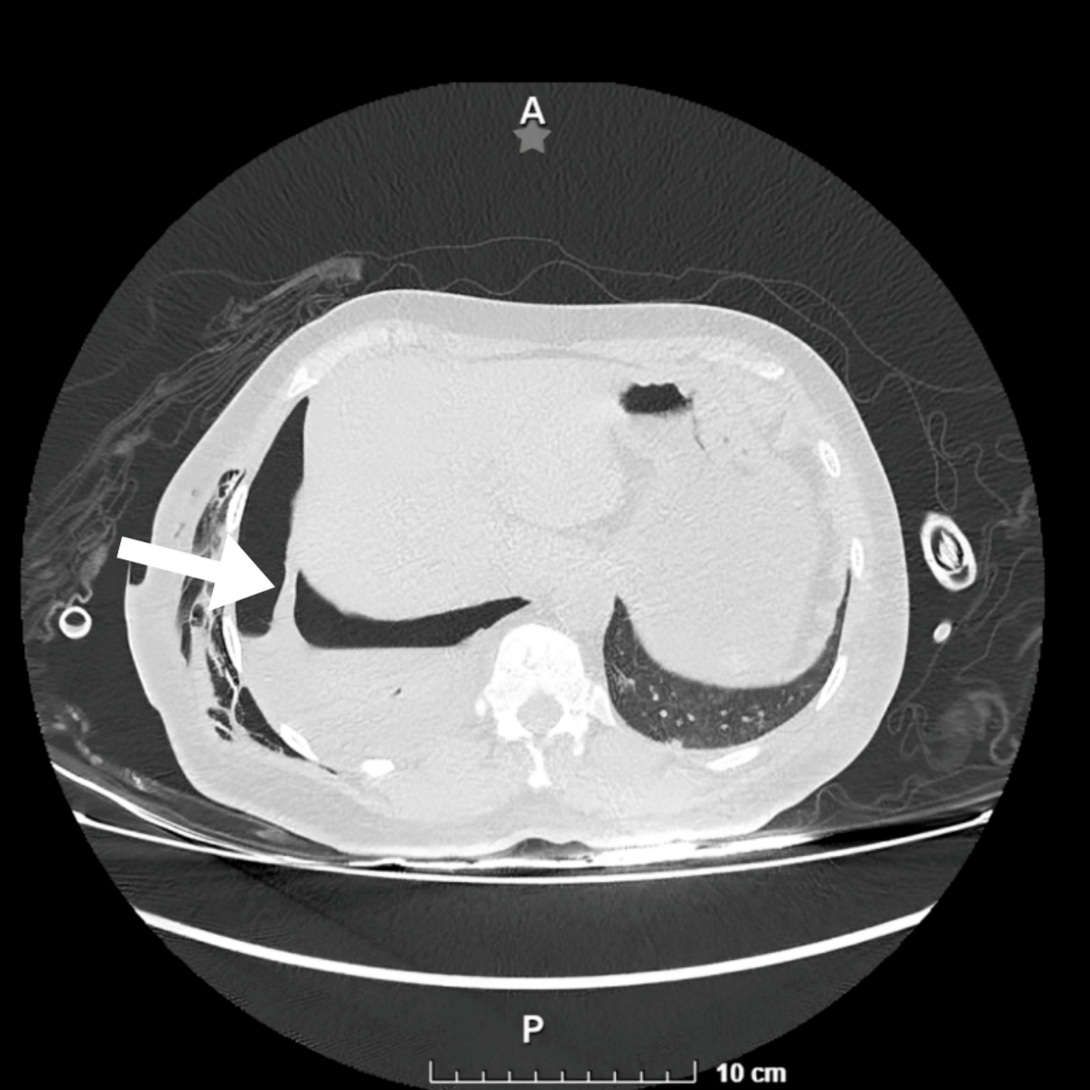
Computed tomography (CT) of the thoracoabdominal area in pulmonary window CT scan showing communication between the pleural and peritoneal cavities in the right hemithorax (white arrow)
